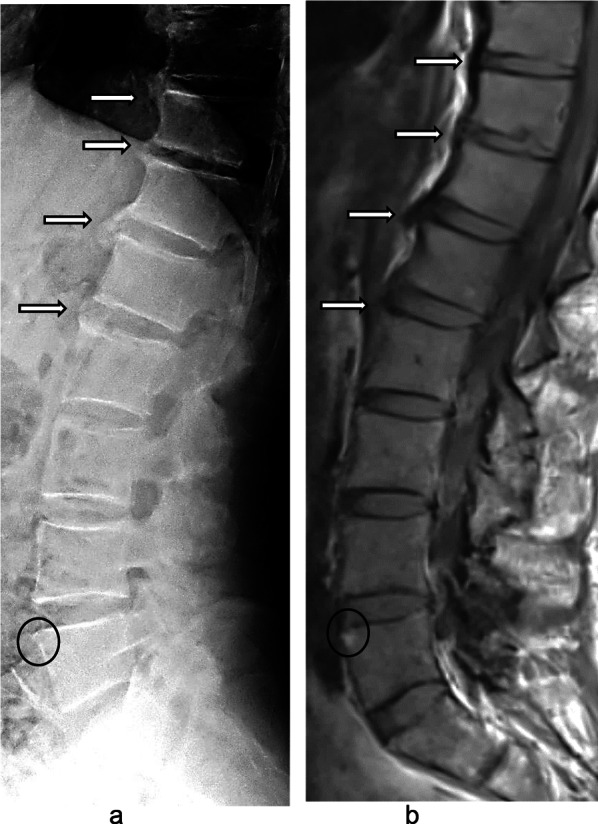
Radiography (a) and T1-weighted MRI (b) of the lower thoracic and lumbar spine. Images in the sagittal plane in the same patient (male, 53 years old) diagnosed with axial SpA. The extent of osseous chronic changes at the anterior vertebral corners is depicted in more detail with radiography than with MRI (white arrows), while a presumed disease-related fatty transformation at the ventral vertebral corner of L5 is only appreciated with MRI (black circles)
